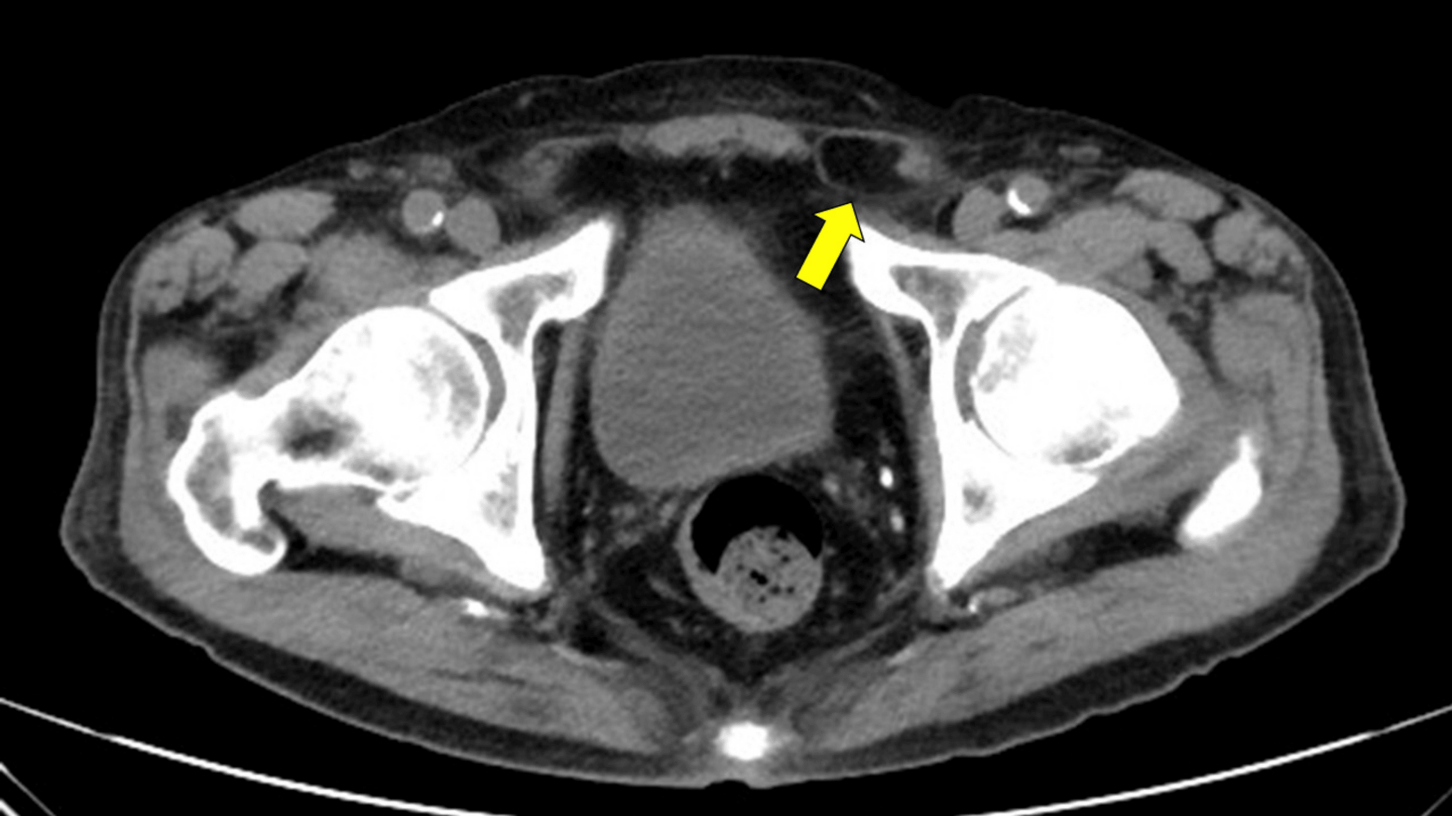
Preoperative computed tomography scan of a left inguinal hernia Preoperative abdominal computed tomography demonstrating a left inguinal hernia (yellow arrow) with protrusion of intra-abdominal fat into the inguinal canal.
